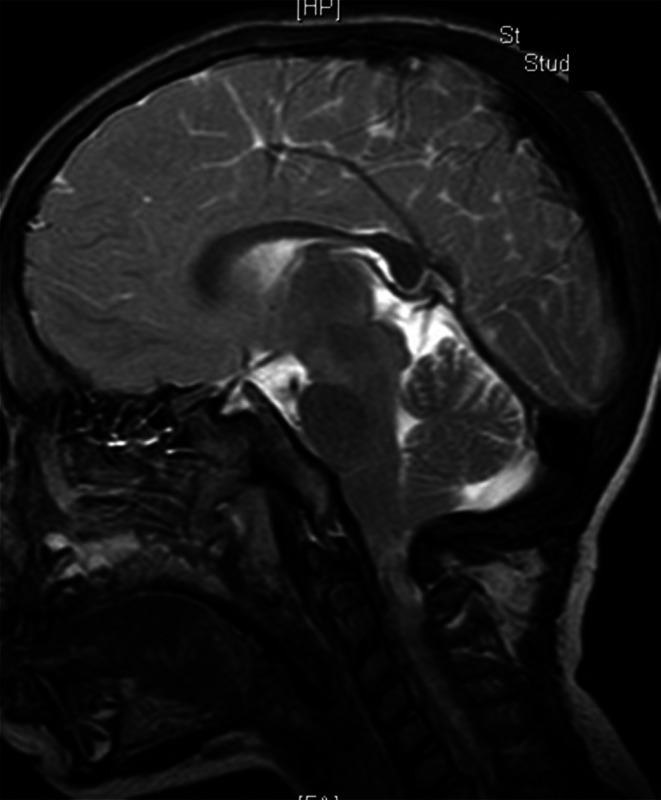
Sagittal view on cranial magnetic resonance imaging. Note dysgenesis of the corpus callosum.
